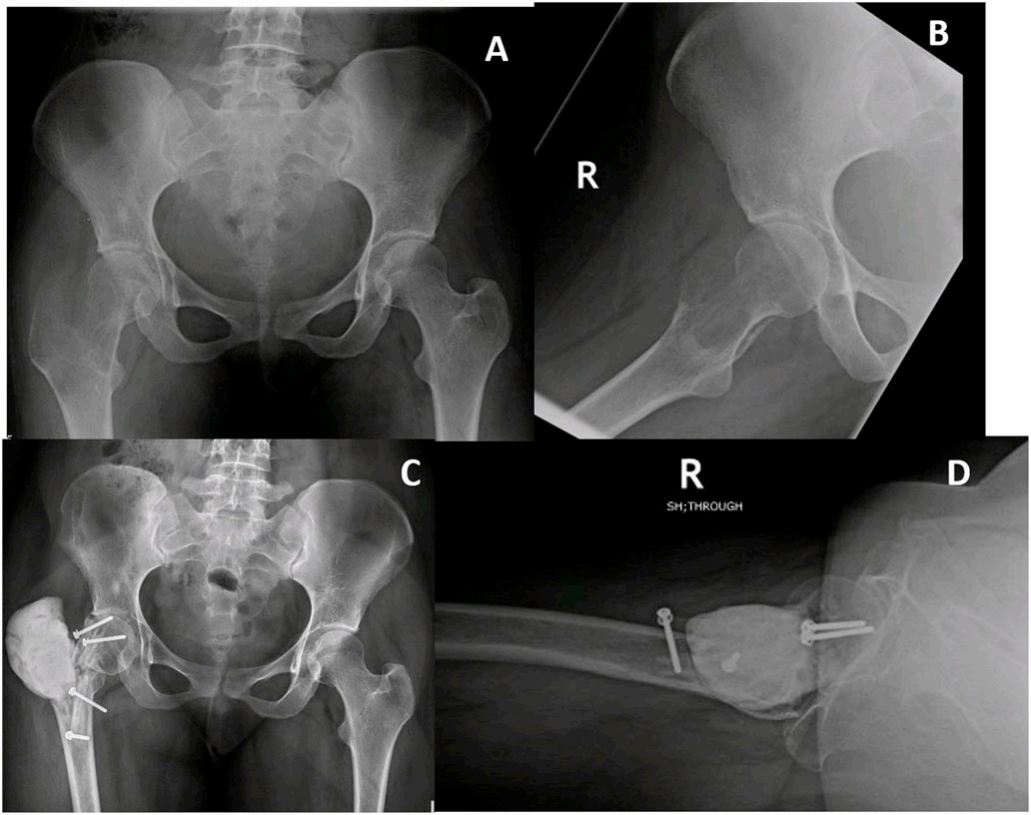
Preoperative (A & B) and postoperative (C & D) X-ray images of GCT proximal femur with salvaging of head of femur.
